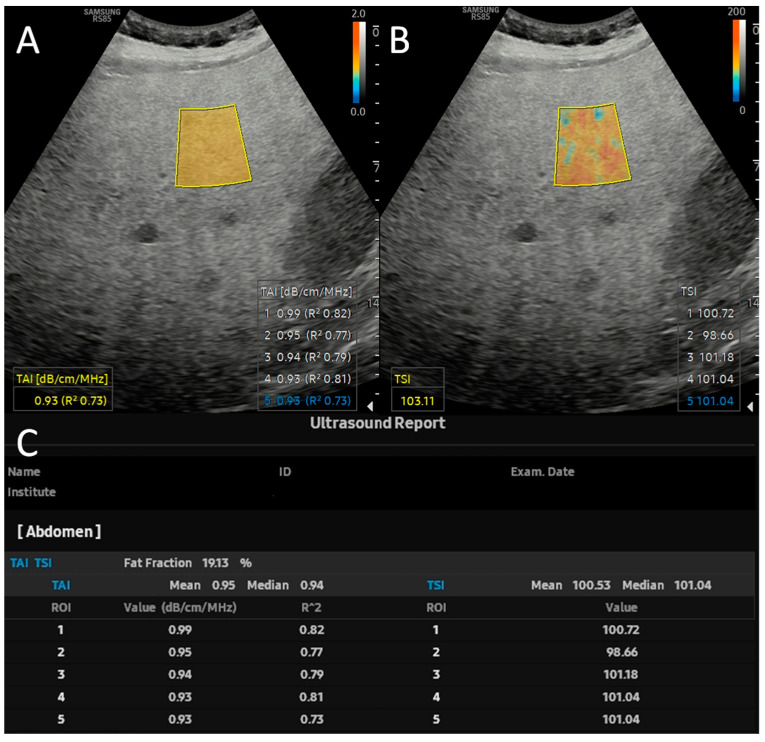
Quantitative ultrasound measurement and calculation of ultrasound fat fraction. (A) The tissue attenuation coefficient (TAI^TM^), equivalent to the attenuation coefficient (AC), and (B) the tissue scatter-distribution coefficient (TSI^TM^), equivalent to the backscatter-distribution coefficient (BSC-D), are measured five times inside a color-coded window placed by the examiner in the right lobe 2 cm from the liver capsule. (C) The software automatically calculated the ultrasound fat fraction (USFF) from five TAI and TSI values and displayed it in the quantitative report.
